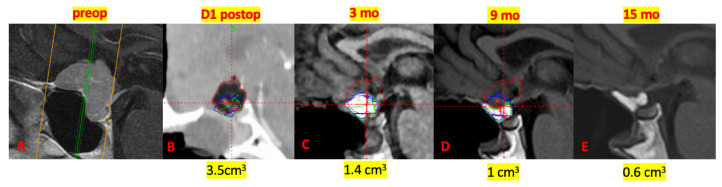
Representative case of fat graft evolution following reconstruction for a tuberculum sellae meningioma. (A) Intraoperative view after tumor resection and reconstruction. (B) Postoperative day 1 CT demonstrating fat graft in situ (volume: 3.5 cm^3^). (C–E) Follow-up MRI showing progressive reduction in graft volume at 3 months (1.4 cm^3^), 9 months (1.0 cm^3^), and 15 months (0.6 cm^3^) (red delineates the fat volume in day 1, blue at 3 months, and green at 9 months).
